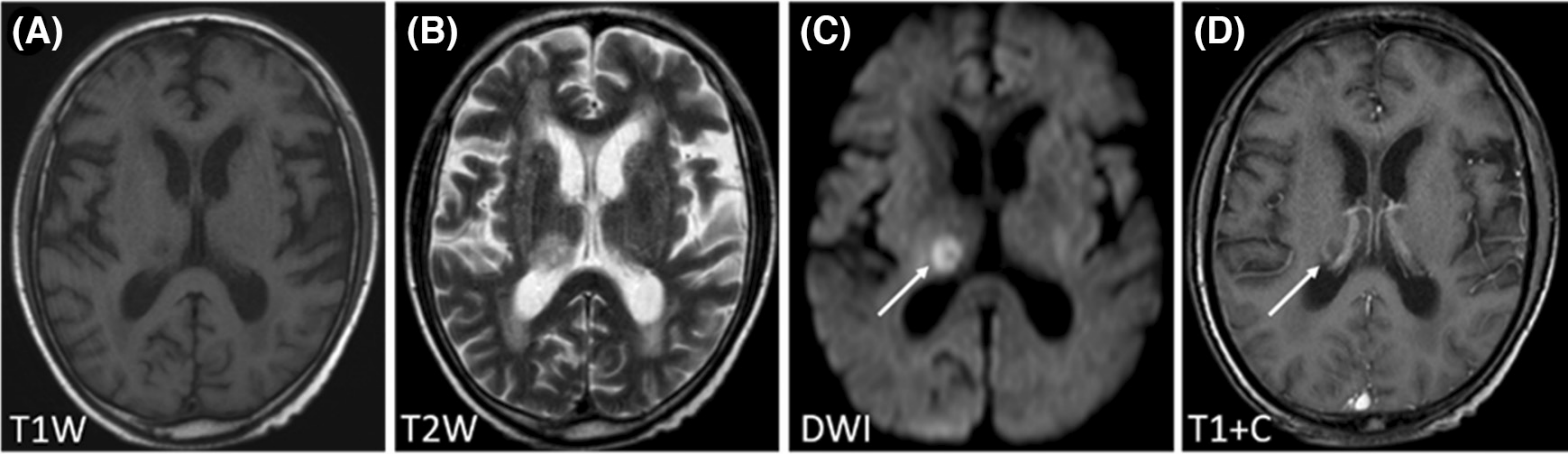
Pattern II of brain metastases in SCLC. Pattern II: A 67‐year‐old man was diagnosed with BMs of SCLC for the first time. The patient had four lesions. One lesion (white arrow) was located in the right thalamus, with hypointensity in T1WI (A), hyperintensity in T2WI and DWI (B, C), and ring enhancement (D). BMs, brain metastases; DWI, diffusion‐weighted imaging; SCLC, small cell lung cancer.
